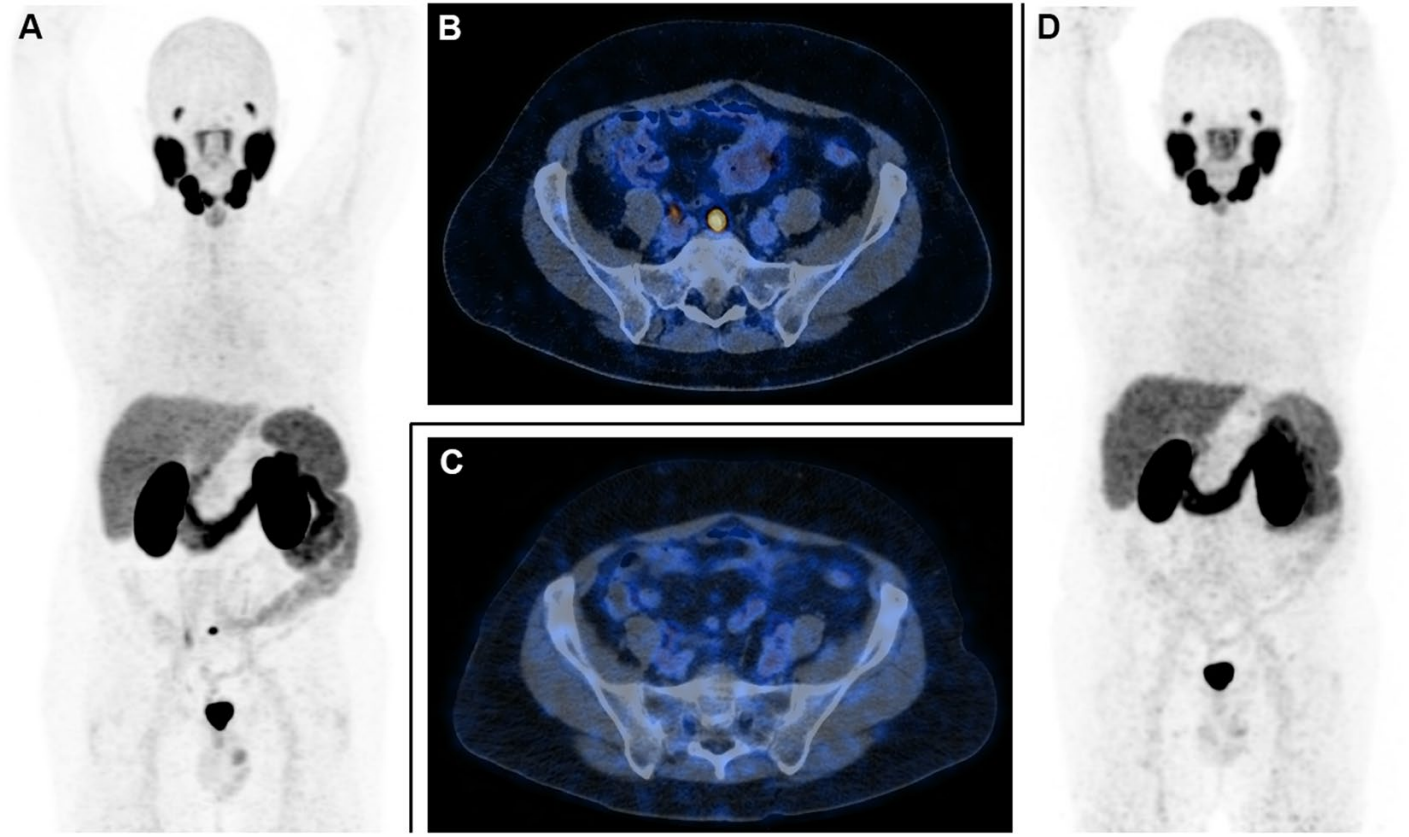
A 61-year-old man initially treated by prostatectomy 6 years before the PSMA-11 PET/CT (ISUP 4, high risk according to d’Amico classification). The first biochemical recurrence occurred 20 months after the surgery and was treated by radiation therapy of the prostatic lodge, which had no effect on the PSA serum level, and androgen deprivation therapy (ADT) by LHRH agonist was initiated (PSA nadir undetectable). (A) Rise in the PSA appeared 3 years after the beginning of ADT. ^18^F-flurocholine PET/CT, which was performed 3 months before PSMA-11 PET/CT, was negative (PSA at 1.3 ng/ml). PSMA-11 PET/CT (PSA at 1.5 ng/ml) demonstrated an isolated malignant focus in a presacral lymph node (A: maximum intensity projection view (MIP); (B): axial fusion slices centered on the pelvis), probably related to prostate cancer. Stereotactic radiation therapy was performed on the presacral lymph node and was effective on PSA (at 0.28 ng/ml stable 12 months after PSMA-11 PET/CT). A second PSMA-11 PET/CT performed 10 months after the first one demonstrated the disappearance of the malignant locus from the presacral lymph node (D: MIP; C: axial fusion slices centered on the pelvis). The ADT regimen was not changed.
